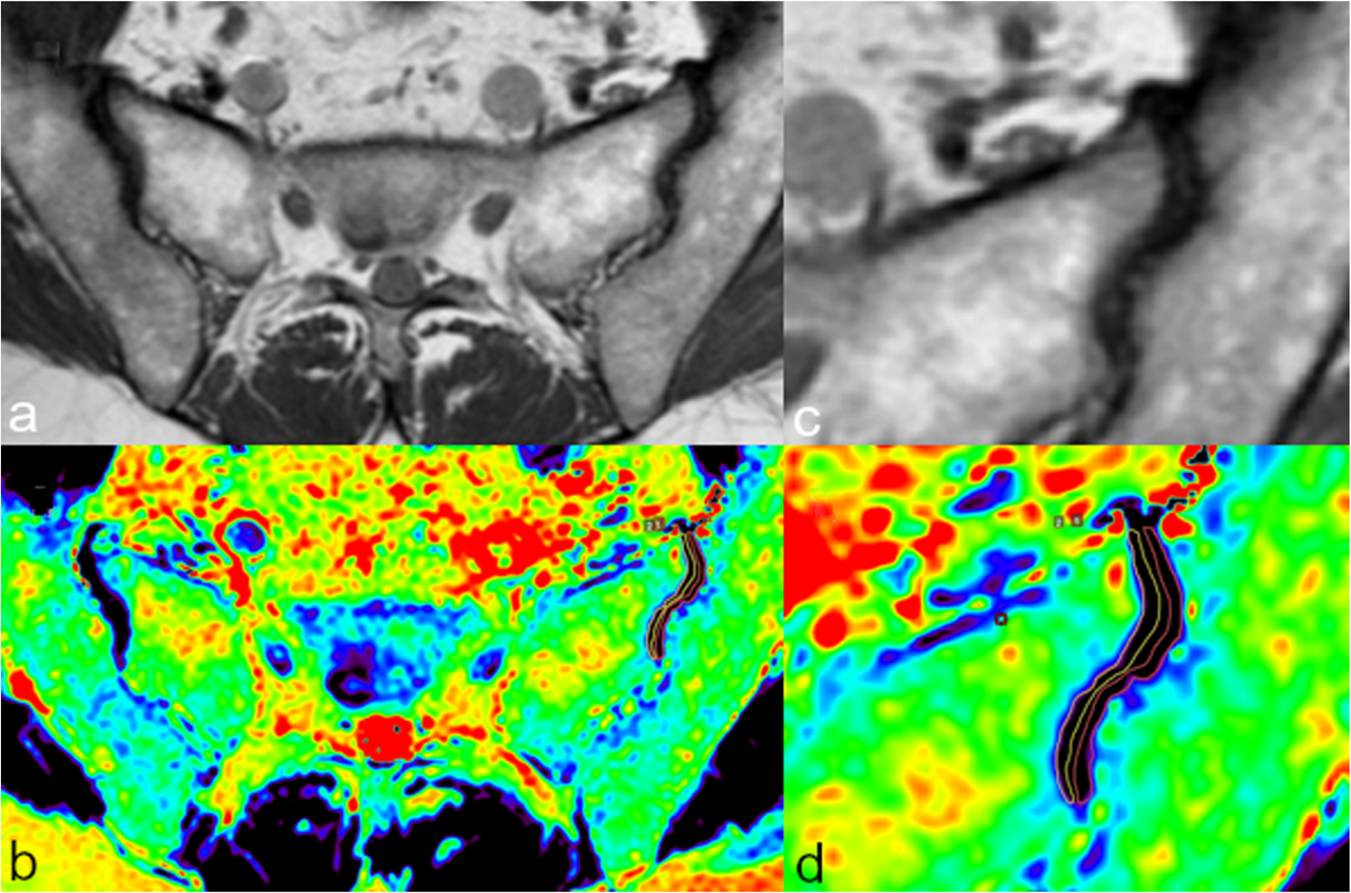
Magnetic resonance imaging of the sacroiliac joints of a 42-year-old female patient. Oblique axial T1-weighted turbo spin-echo image of both sacroiliac joints (a); in detail the left sacroiliac joint (c). The corresponding oblique axial T2 maps (b, d) show the ROIs manually drawn on both sacral and iliac articular side of the joint space of the left sacroiliac joint
